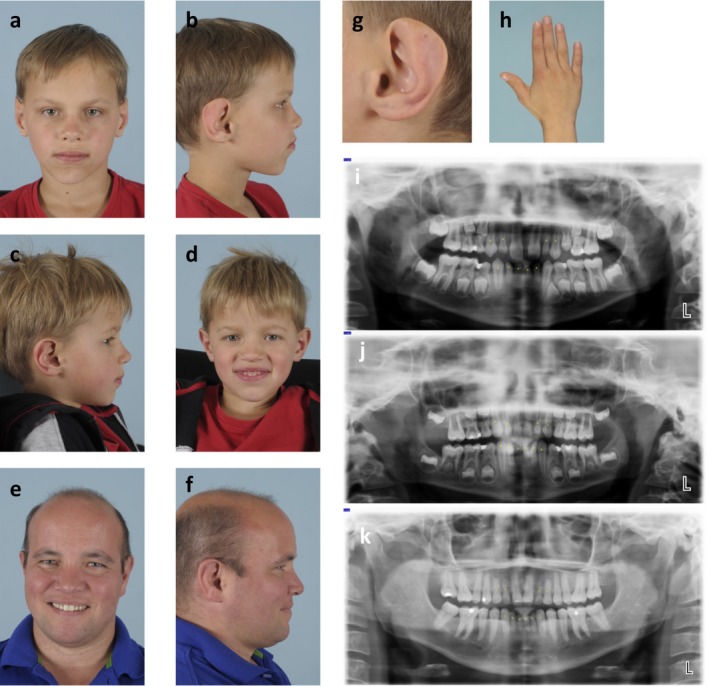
(a) Index patient (proband III‐5). (b) Index patient (proband III‐5). (c) Proband III‐6. (d) Proband III‐6. (e) Proband II‐3. (f) Proband II‐3. (g) Left ear of the index patient, note the mostly absent helix. (h) Slight underdevelopment of the thumb of the index patient. (i) Dental panoramic radiograph of the index patient. Yellow dots indicate deciduous teeth. (j) Dental panoramic radiograph proband III‐6. Yellow dots indicate deciduous teeth. (k) Dental panoramic radiograph proband II‐3. Yellow dots indicate deciduous teeth
